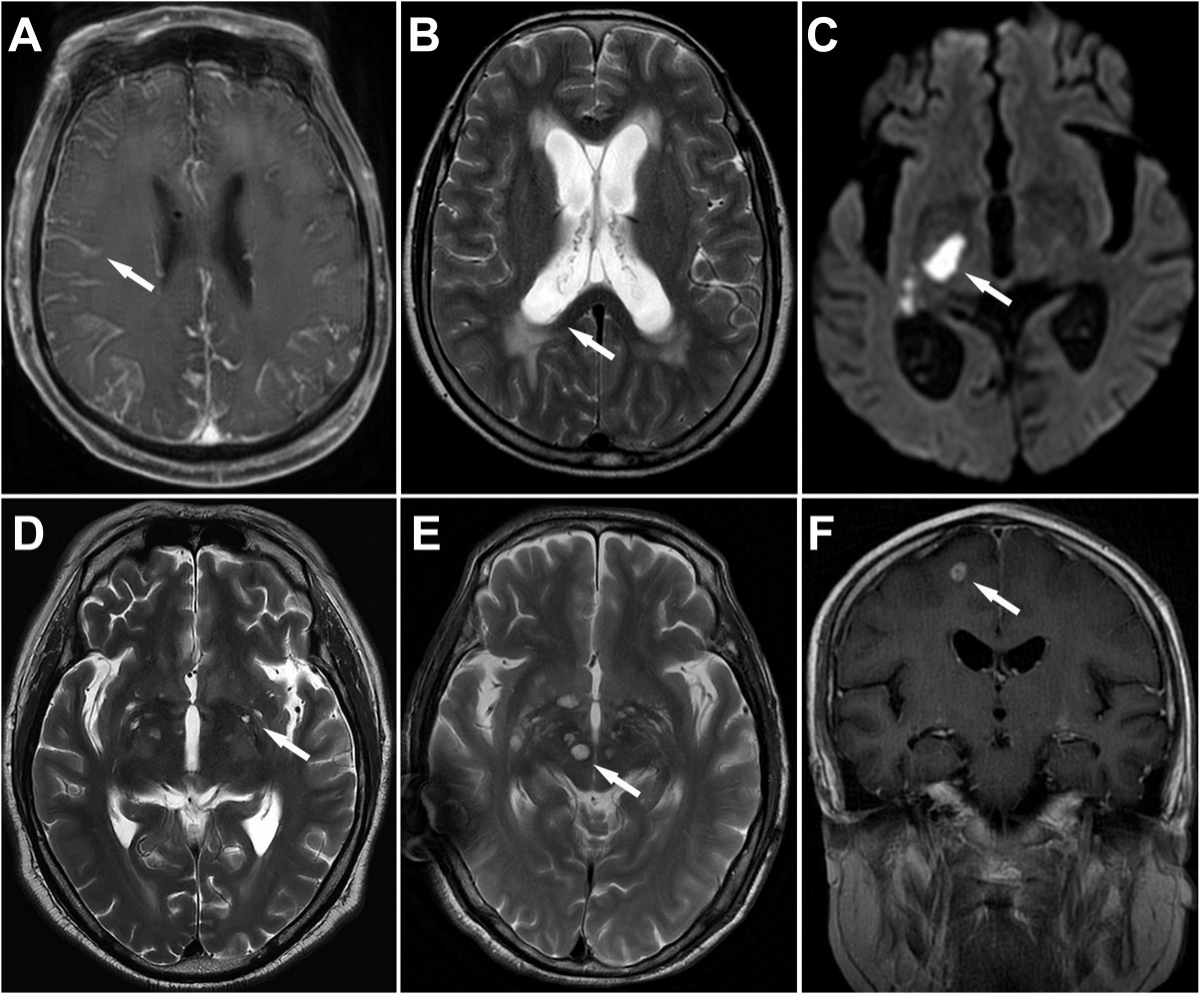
Representative MRI findings in elderly patients with cryptococcal meningitis. (A) Gadolinium contrast-enhanced T1WI shows meningeal enhancement (arrows). (B) T2WI shows hydrocephalus (arrows). (C) DWI shows a acute cerebral infarct (arrows). (D) T2W1 shows basal ganglia lesions of Virchow-Robin spaces dilatation (arrows). (E) T2W1 shows basal ganglia lesions of gelatinous pseudocysts (arrows). (F) Gadolinium contrast-enhanced T1WI shows cryptococcoma (arrows). DWI = Diffusion-weighted image; MRI = magnetic resonance imaging; T1WI = T1-weighted image; T2WI = T2-weighted image.
